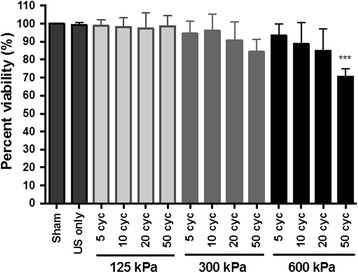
Cell viability 24 h post-sonoporation treatment. Here, we observe decreasing cell viability with increasing pulse length and pressure. As expected, ultrasound exposure in the absence of PCCAs does not affect cell viability. Furthermore, the PCCAs themselves did not have a toxic effect on cells as evidenced by the high viability in treatment groups below the PCCA activation threshold (i.e., 125 kPa groups). One-way ANOVA was used followed by Dunnett’s multiple comparisons test to compare each treatment to the sham control. p* ≤ 0.001
